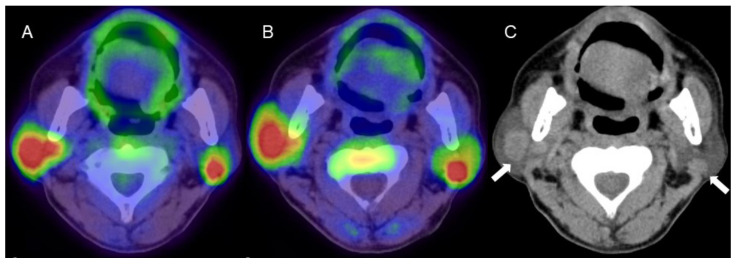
A man in his 60s with pathologically proven Warthin’s tumor in the parotid glands bilaterally. (A) Fused FDG-PET/CT image (axial view), (B) fused 4DST PET/CT image (axial view), and (C) plain CT (axial view). Intense uptake of both FDG (SUV_max_: 9.6) and 4DST PET/CT (SUV_max_: 12.0) is seen in bilateral nodular lesions, which show high intensity on plain CT (arrows) consistent with the typical features of Warthin’s tumors. Aspiration biopsy revealed oncocytes and abundant lymph-node cells and epithelium with eosinophilic cytoplasm, and a diagnosis of Warthin’s tumor was made based on pathological findings.
